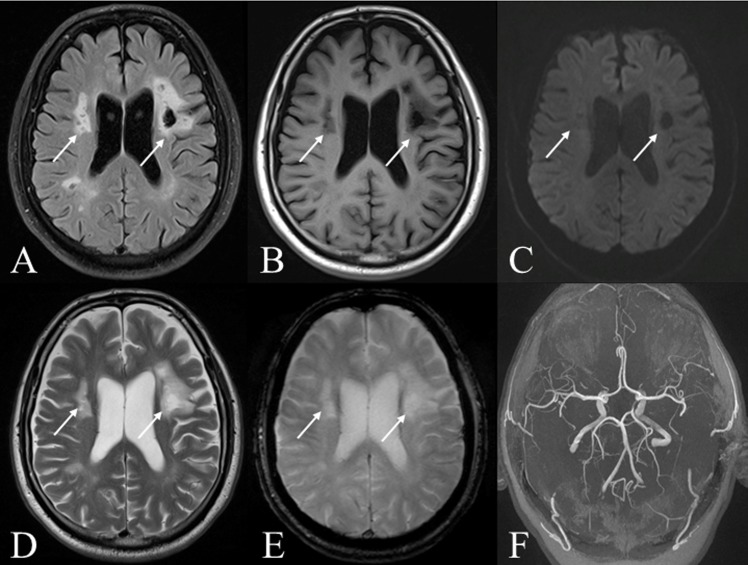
MRI follow-up six years after nonperimesencephalic subarachnoid hemorrhage of a 59-year-old male patient (Table 1, #15). DCI-related lesions are indicated by arrows. *A-E* show bilateral chronic liquor-isointense areas with surrounding gliosis and no diffusion restriction, nor hemosiderin residues. *F* depicts no aneurysm in TOF-angiography. *Sequences*: *A =* T2 TIRM weighted, *B* = T1-TIRM weighted, *C* = diffusion-weighted, *D* = T2 weighted, *E* = T2* weighted, *F* = time-of-flight-angiography.
